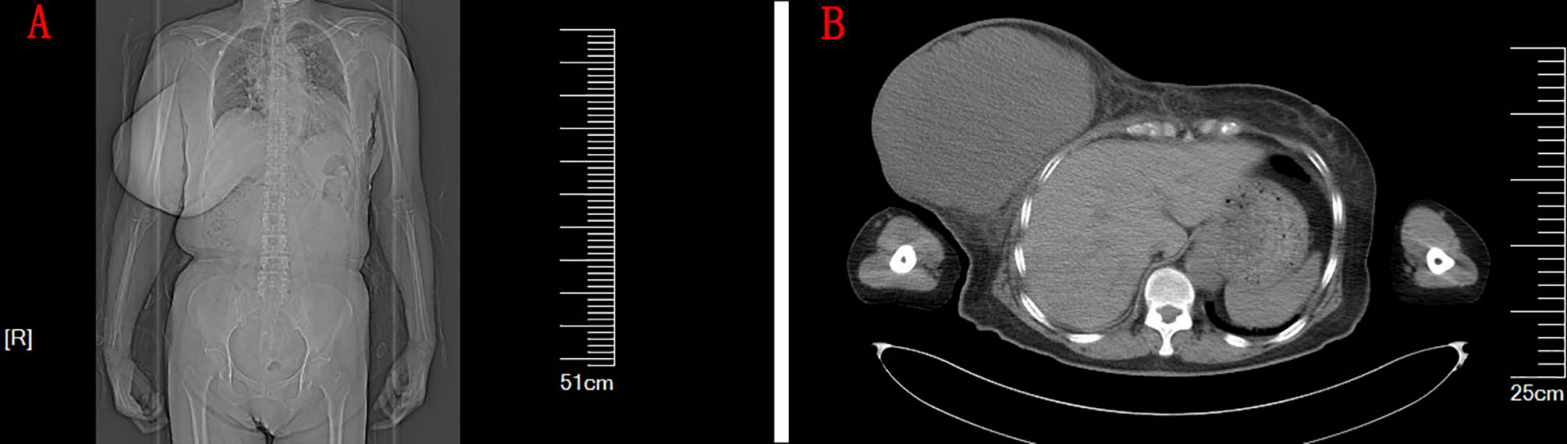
(A) The CT image of the patient’s chest and abdomen. (B) The CT of the chest. A large soft tissue density mass was seen in the right breast, measuring approximately 17.1 cm × 13.2 cm × 17.2 cm, with clear borders, regular morphology, and uniform density.
